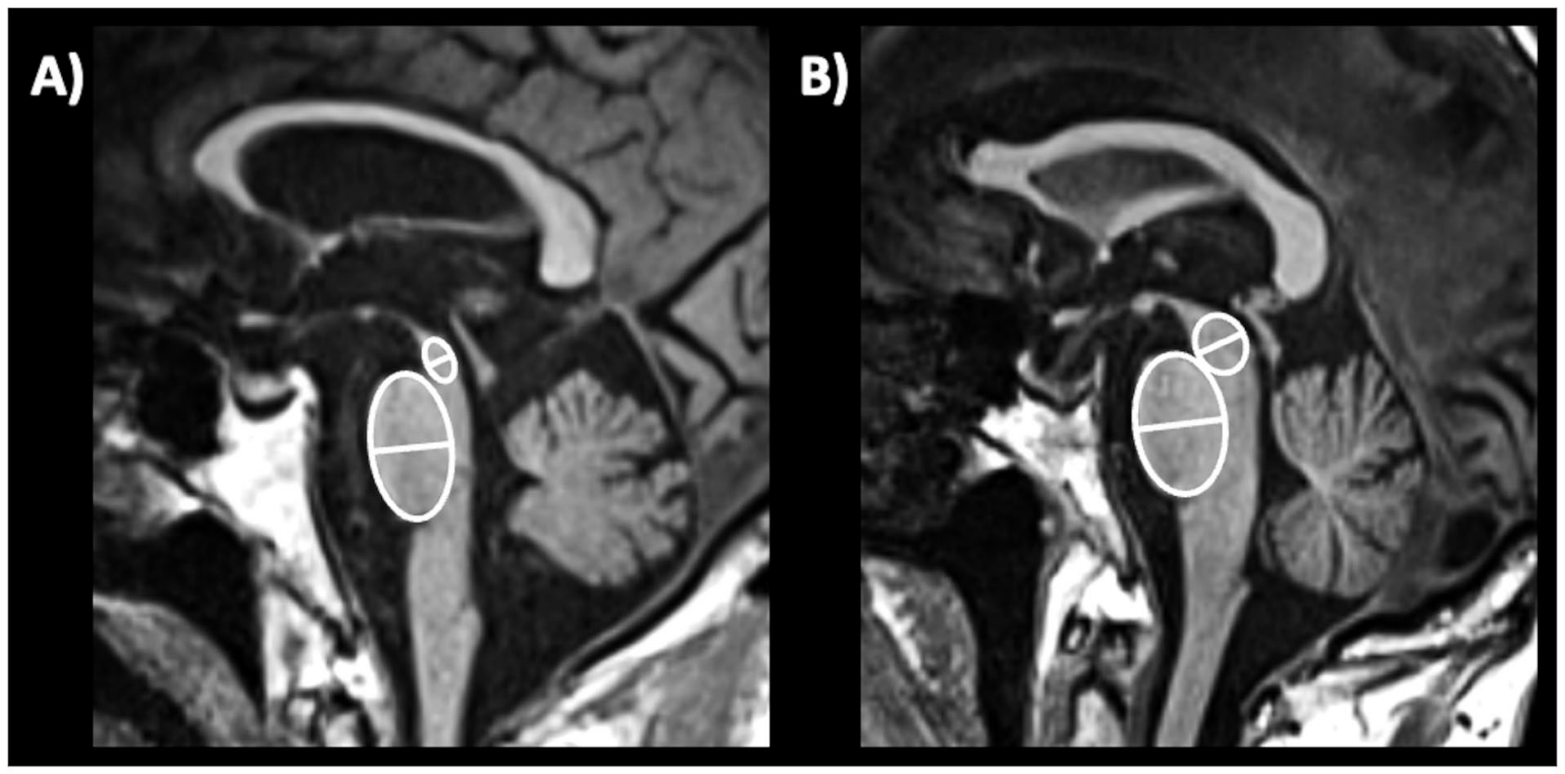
A 3T brain T1-weighted midsagittal MR image showing how to measure the midbrain and pons diameters, as described by Massey et al. (221) in a patient with progressive supranuclear palsy (A) and in a patient with Parkinson’s disease (B).
